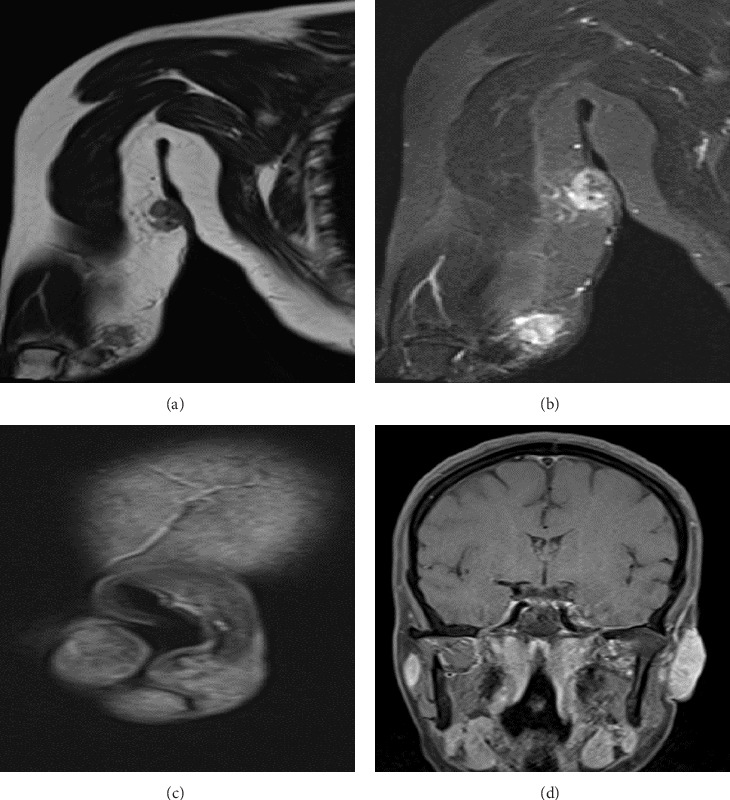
(a–d) MRI of the right arm showed a well-defined T1W hypointense (a) and T2W heterogeneous hyperintense (b) soft tissue lesion with adjacent cystic spaces in the mid 1/3rd of the arm and near the olecranon process. MRI of the face showed that the left ear pinna is swollen and oedematous with diffuse T1/FS hyperintense (c) and T2/TIRM heterogeneous hyperintense (d) soft tissue showing diffusion restriction and intense contrast enhancement.
